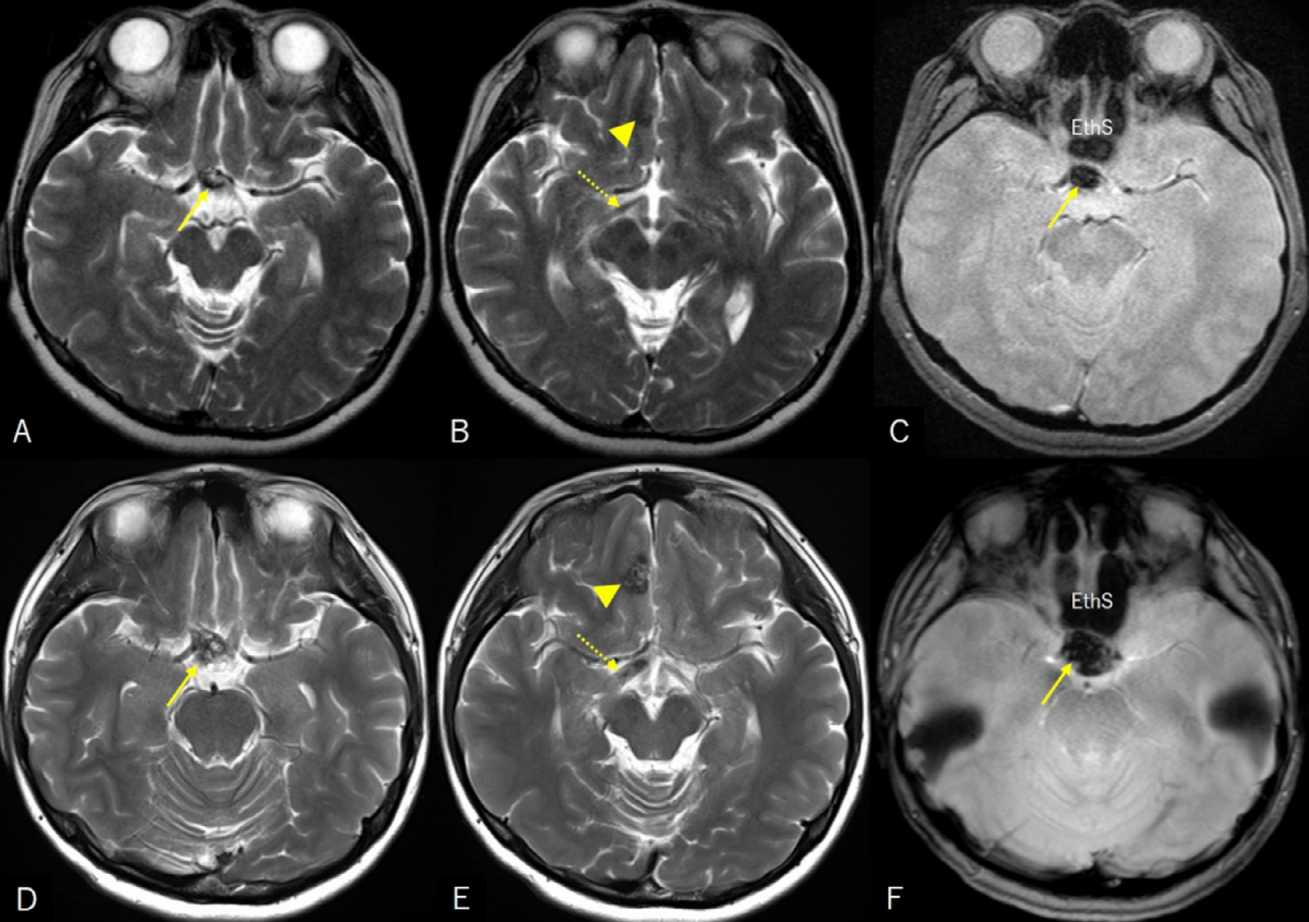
(A-C) Axial T2- (A, B) and gradient-echo T2*-weighted (C) magnetic resonance images performed 10 months before presentation showing a low intense mass in the suprasellar region (A, C, arrow), in addition to a cavernous malformation in the right frontal lobe (B, arrowhead). The right optic tract appears intact (B, dashed arrow). (D-F) Axial T2- (D, E) and gradient-echo T2*-weighted (F) magnetic resonance images at the presentation showing a marked enlargement of the suprasellar mass and swelling of the right optic tract. Cavernous malformation in the right frontal lobe does not show any signs of recent hemorrhage (E, arrowhead). A, C, D, and E are images near the same level. EthS, ethmoid sinus.
